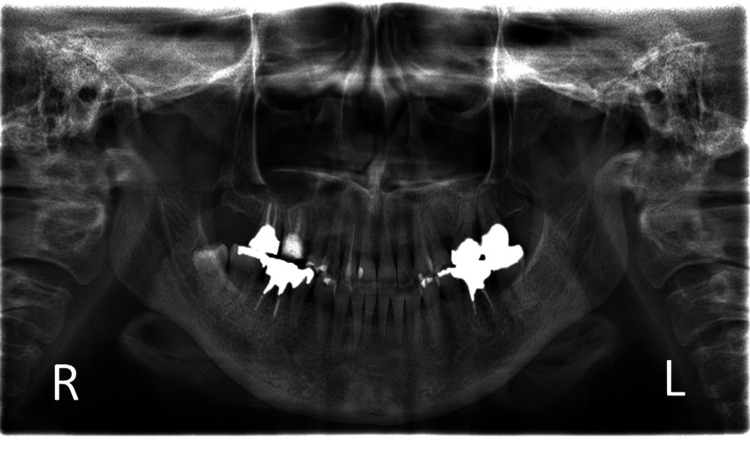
Panoramic radiographs Panoramic radiographs revealed a slight radiolucent area at the palatal root apex of the upper right first molar, but no alveolar bone resorption or other abnormal findings.
